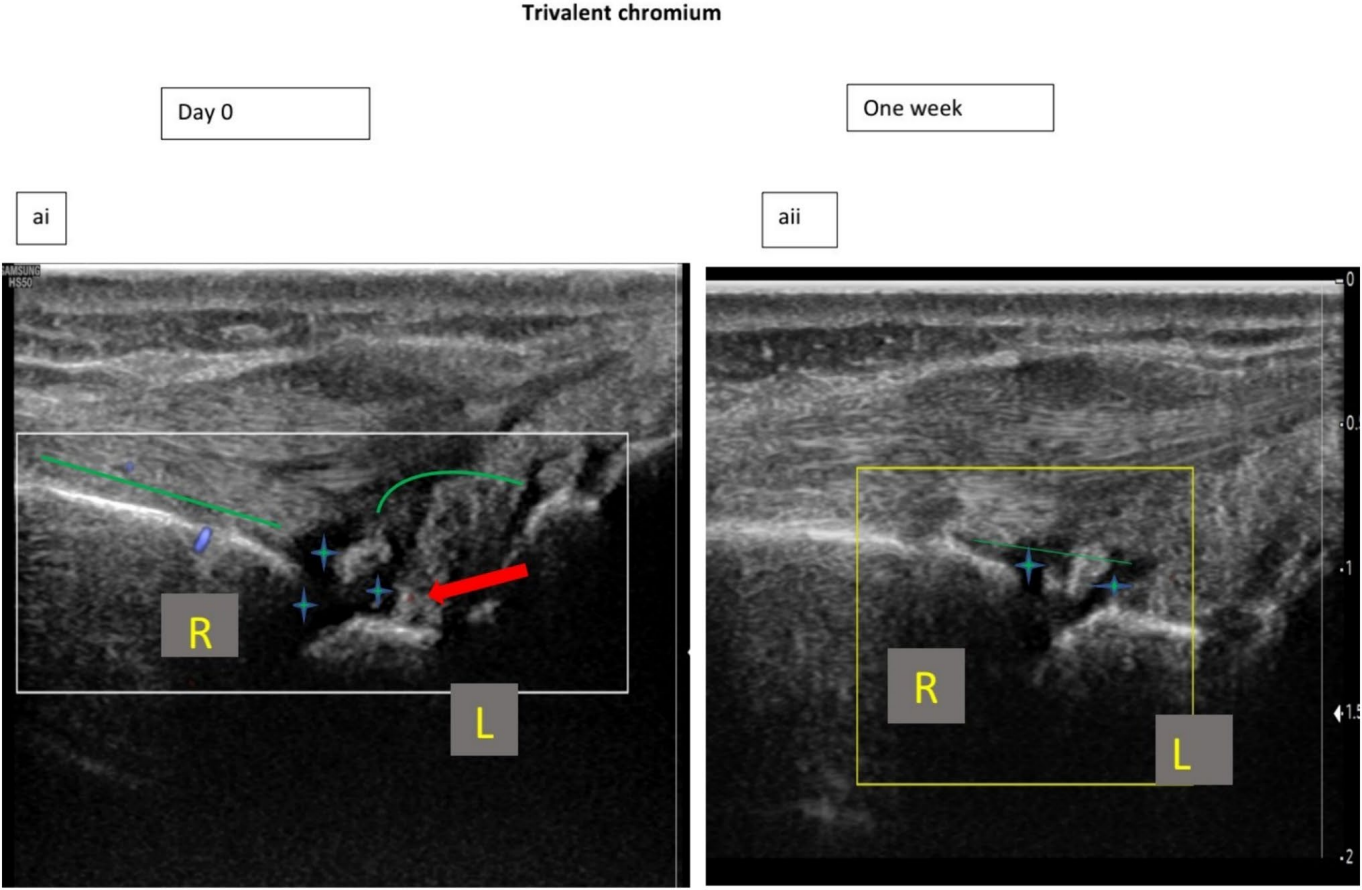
The hands ultrasound of one patient who received Cr III for 1 week, (i)Hands ultrasound in the first visit revealed that : SH : grade 3 (green line), PDUS : grade 2 in the right radio-lunate and left fifth MCP joints (red arrow PDUS = 1 in radio-lunate joint (RL) joint) and blue sign indicates the effusion in the same joint. Ii) after 1 week of Cr III intake, the ultrasound was done on day 11 and showed that: SH decreased to be of grade 2, PDUS in both joints disappeared = 0, effusion decreased
